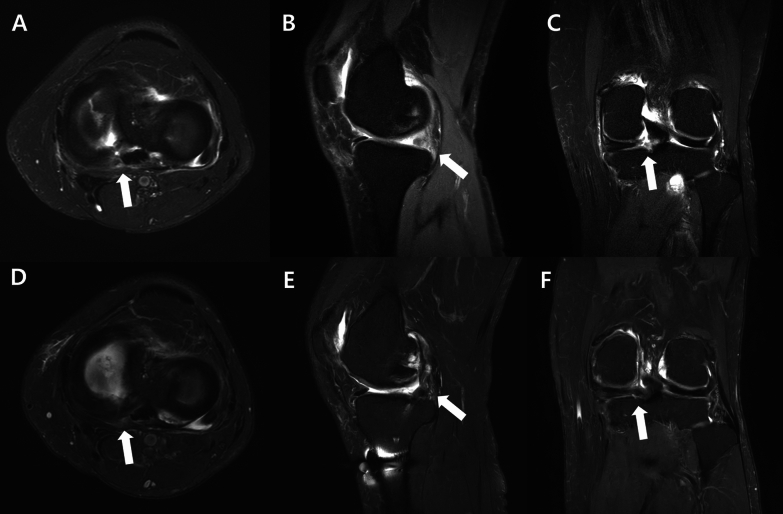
Preoperative and 1-year postoperative MRI scans of a patient who underwent posterior approach repair for a medial meniscus posterior root tear in the left knee. White arrows indicate the tear site in images A–C, and the healed root in images D–F. A Preoperative axial image; B preoperative sagittal image; C preoperative coronal image showing the medial meniscus posterior root tear. D One-year postoperative axial image; E sagittal image; and F coronal image demonstrating complete healing with normalization of meniscal signal
